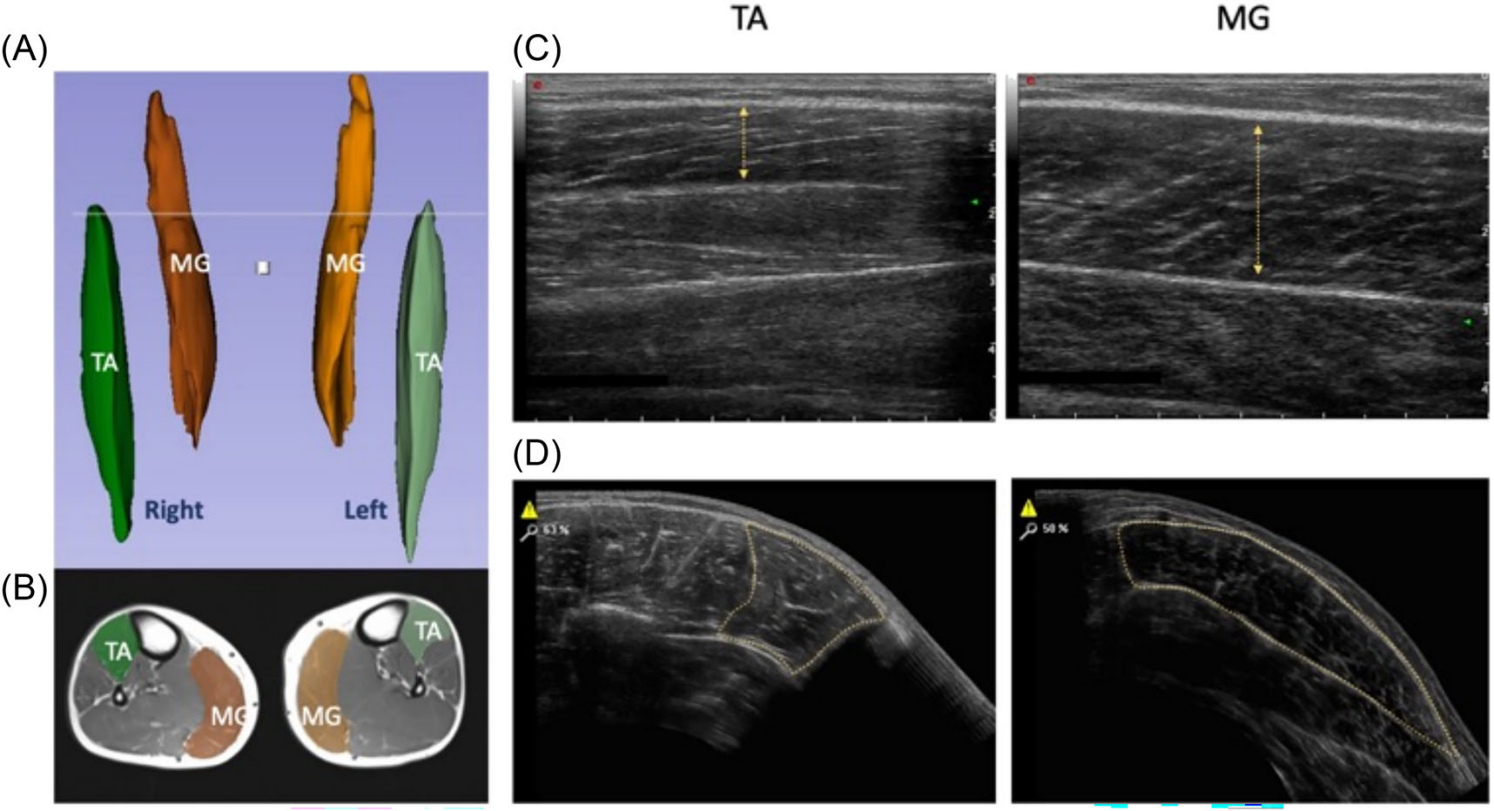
(A) MRI image of tibialis anterior (TA; green) and medial gastrocnemius (MG; orange) and representative 3D segmentation volume analysis. (B) MRI image with representative cross‐sectional area (CSA). Representative ultrasound images of TA (C) and MG (D) with muscle thickness and CSA analysis shown.
